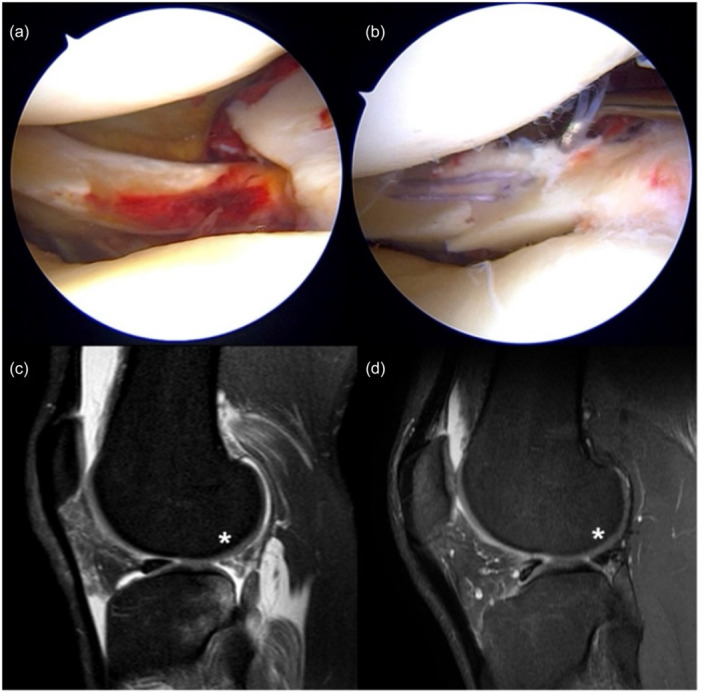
Radial tear of the lateral meniscus posterior horn repair. Arthroscopic and MRI findings demonstrating a lateral meniscus oblique radial (LMOR) tear (a, c), repaired using the all‐inside technique (b). Postoperative Follow‐up MRI demonstrates complete healing (d). MRI, magnetic resonance imaging.
